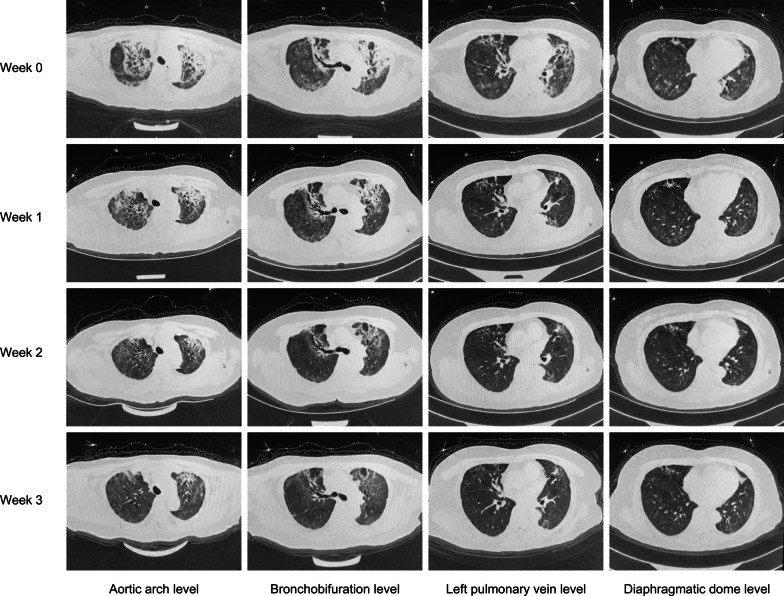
The computed tomography findings of one patient (No. 6) before (week 0) and 1, 2, 3 weeks after HCQ administration, respectively. After the comprehensive treatment together with HCQ, the ground-glass opacity lesions were largely absorbed, while some of the fibrosis stripe lesions were left
